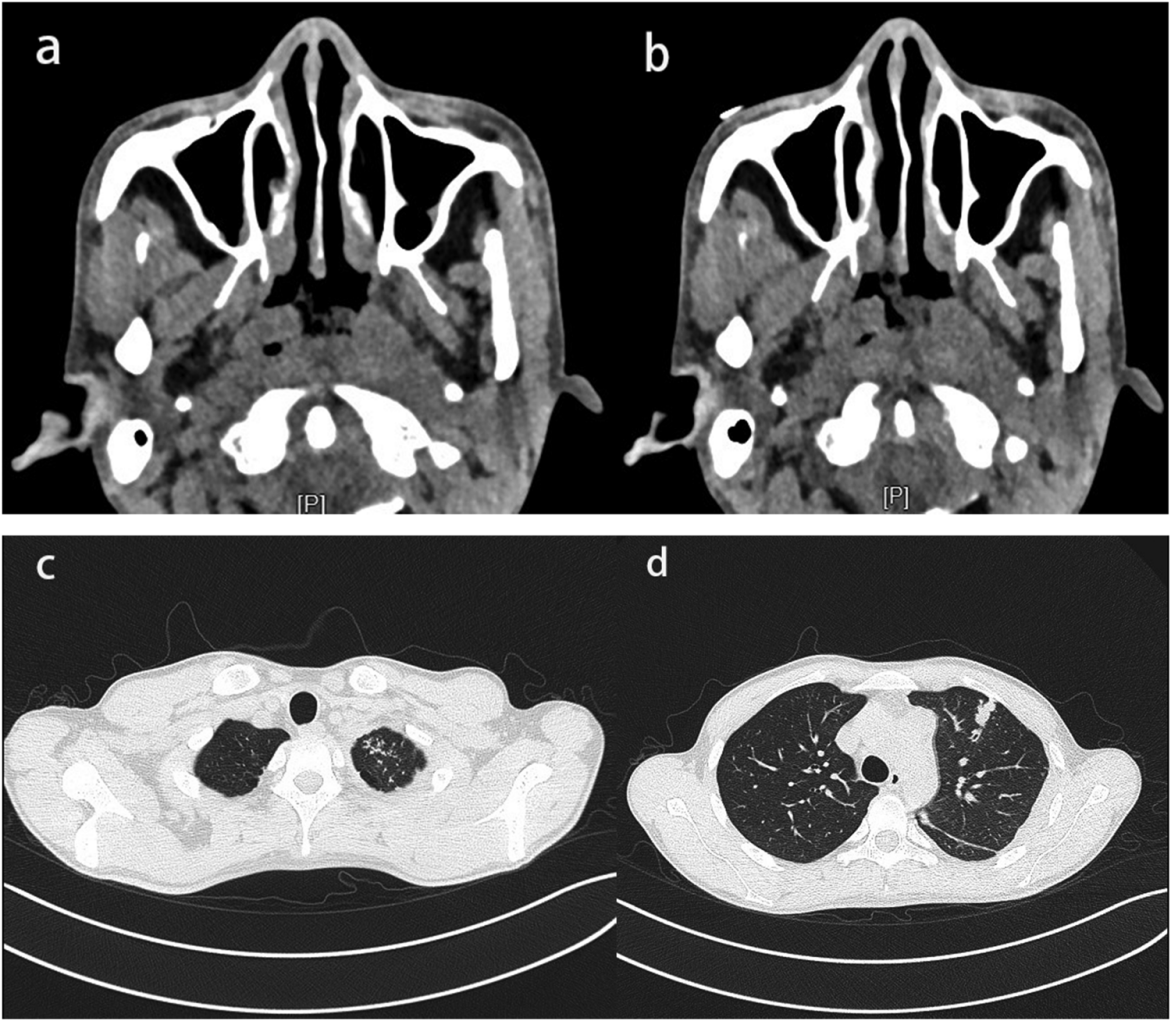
(a and b) Computed tomography of the nasopharynx showing thickening of the mucosa on the right and left side walls and posterior wall with unclear margins. (c and d) Axial computed tomography images of the chest demonstrating centrilobular nodules with tree-in-bud opacification involving apical and anterior segments of the left upper lobe. And showed left-sided pleural thickening.
